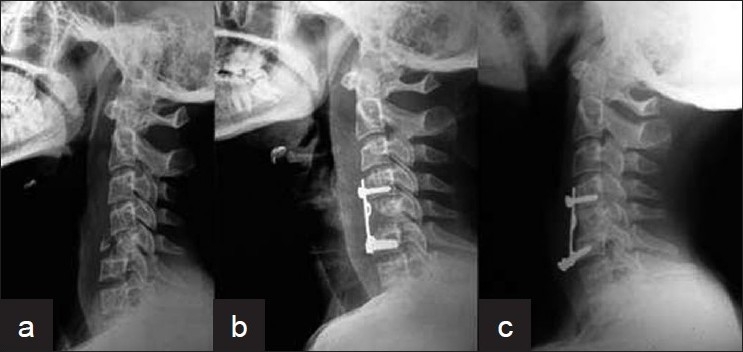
X-ray cervical spine lateral view showing (a) Patient with fracture C5 with retrolisthesis. (b) Post-operative X-ray of the patient treated with anterior corpectomy, fusion, and fixation. (c) Follow up film shows good fusion, inferior screws have backed out, and graft height is reduced
